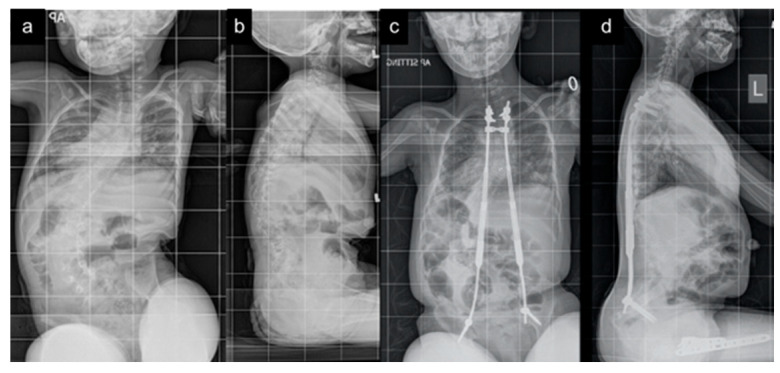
Five-year-old female MCGRs patient with EOS secondary to GMFCS V cerebral palsy. Patient underwent only one surgery for implantation and had magnetic lengthenings in clinic every three months. No complications. Pre-operative EOSQ-24 total score of 45/100 and four-year follow-up score of 57/100. (a) Pre-operative anteroposterior radiograph demonstrating 76-degree scoliosis. (b) Pre-operative lateral radiograph. (c) Four-year post-implantation anteroposterior radiograph. (d) Four-year post-implantation lateral radiograph.
